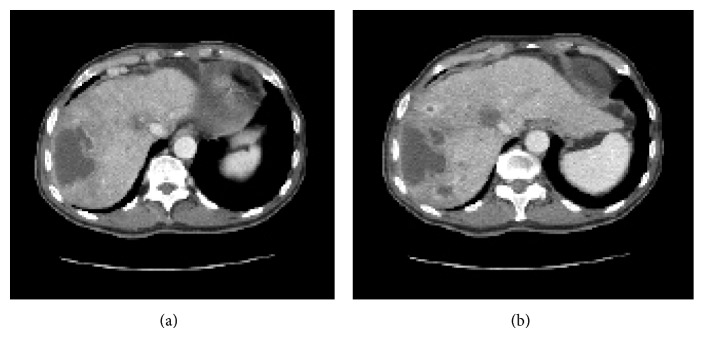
CT image at 28 d after TACE with DC beads. (a) CT image showing a huge abscess in the right lobe of the liver. (b) Many small hypodense areas are located mostly in segments 4, 6, 7, and 8 of the liver.
